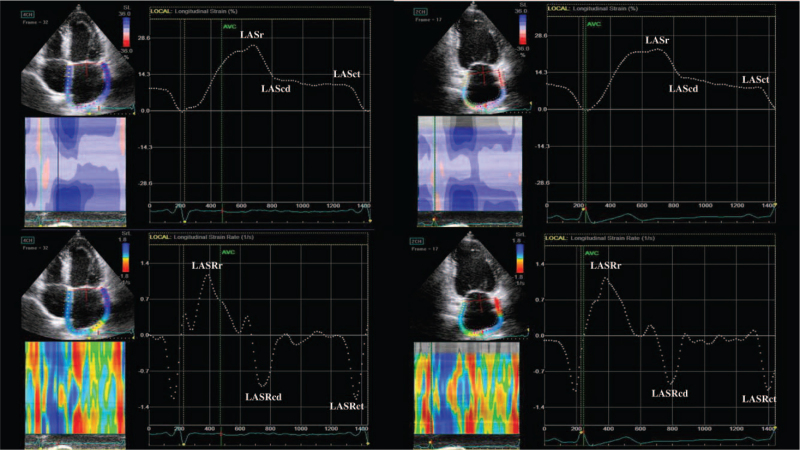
Measurement of peak longitudinal left atrial strain (LAS) and strain rate (LASR) during the reservoir (r), conduit (cd) and contractile (ct) phases obtained from the apical 4 chamber (left panel) and 2 chamber (right panel) views.
